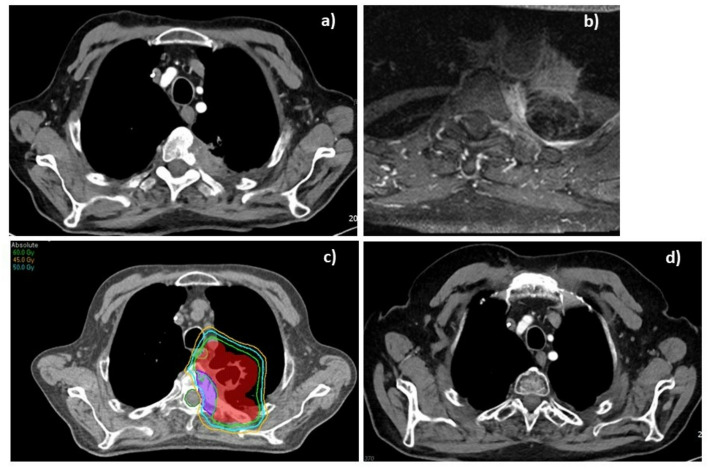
a Contrast-enhanced axial computed tomography (CT) and b magnetic resonance imaging of a patient with locally advanced non-small lung cancer patients invading the spine before radiotherapy (RT); c RT planning with isodose 45 Gy, 50 Gy and 60 Gy, and d CT imaging after RT in the follow up obtaining good response
